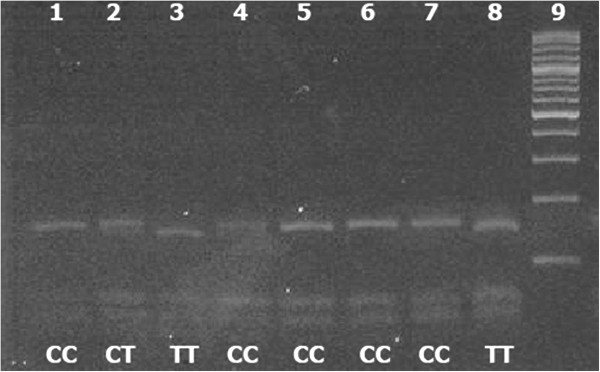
Genotypes generated from 202 bp PCR product acquired from 8 individuals using spasific primers after digested with Tru I restriction enzyme. Lane 2: CT heterozygote; lanes 1, 4, 5, 6, 7: CC genotype; lanes 3, 8: TT genotype; lane 9: 100 bp marker.
